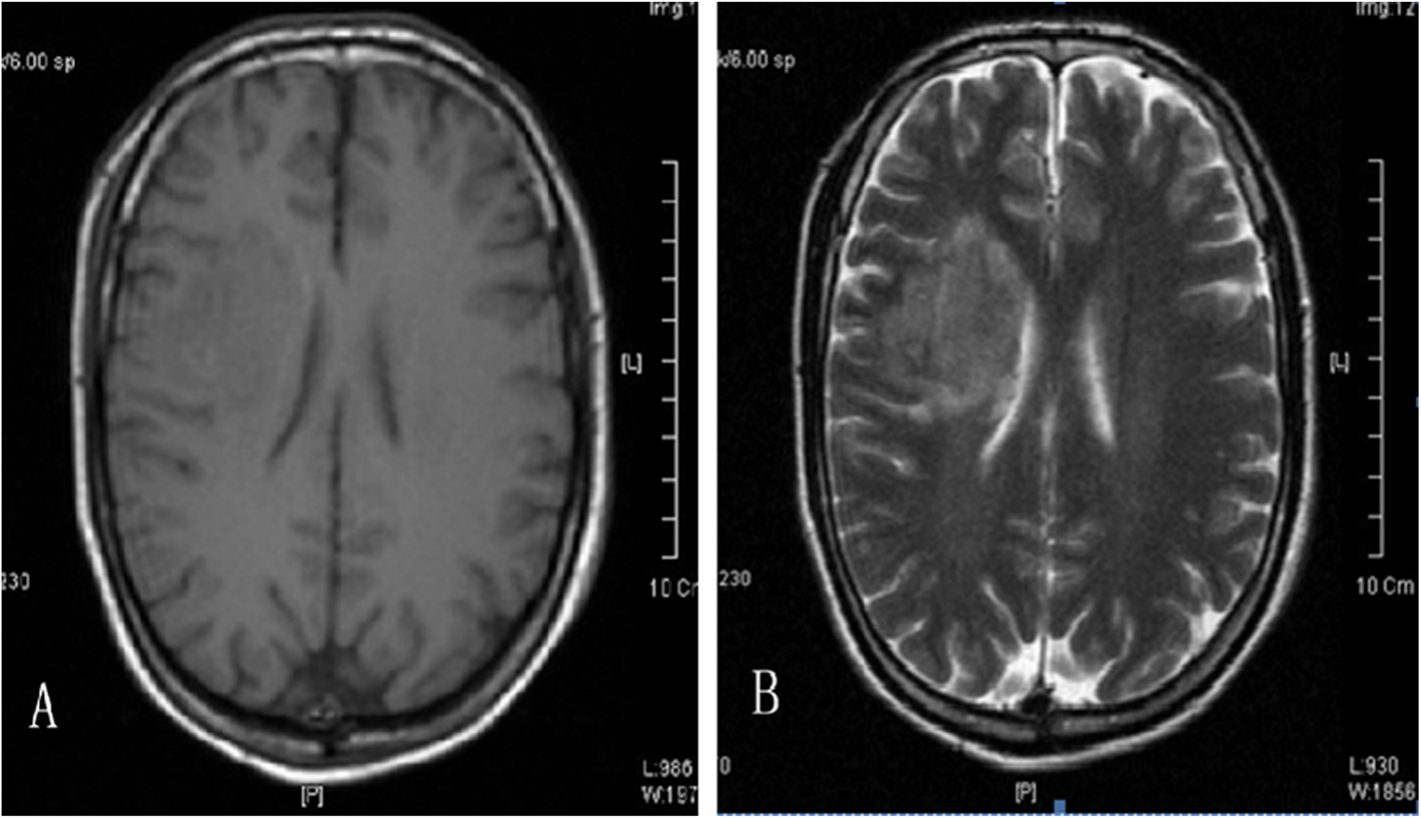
A. MRI (T1-weighted image) showed right basal ganglia cerebral ischemic lesions. B. MRI (T2-weighted image) showed right basal ganglia cerebral ischemic lesions.
